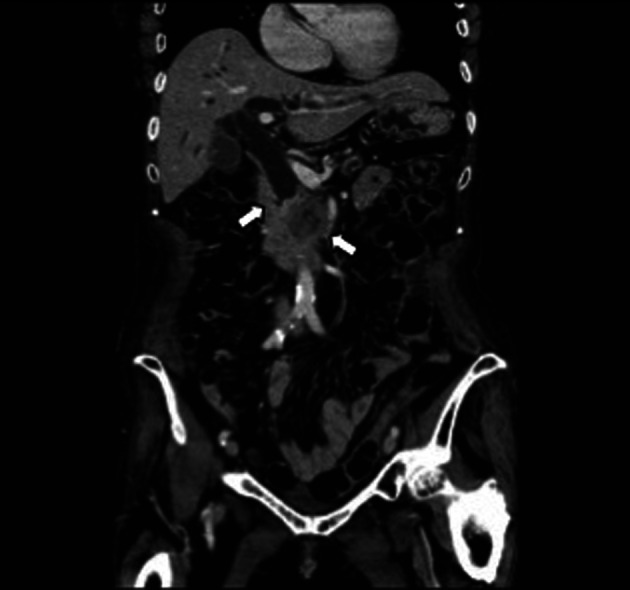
Abdominal CT revealed distal biliary obstruction, and a lesion approximately 3 cm diameter mass lesion located at the head of the pancreas at the first diagnosis (arrow).
